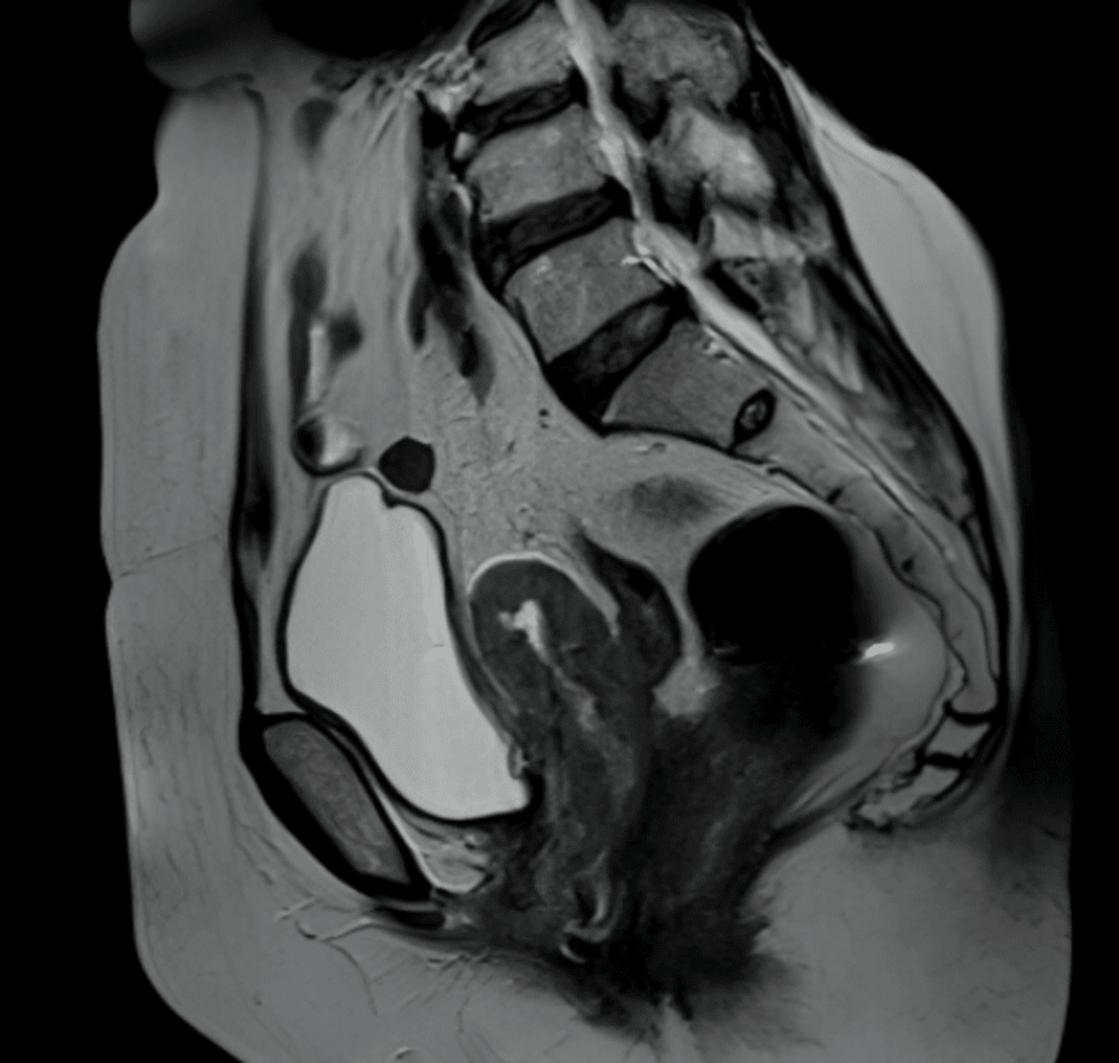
T2-weighted imaging MRI (sagittal view) showing rectal and upper vaginal invasion by cervical carcinoma
